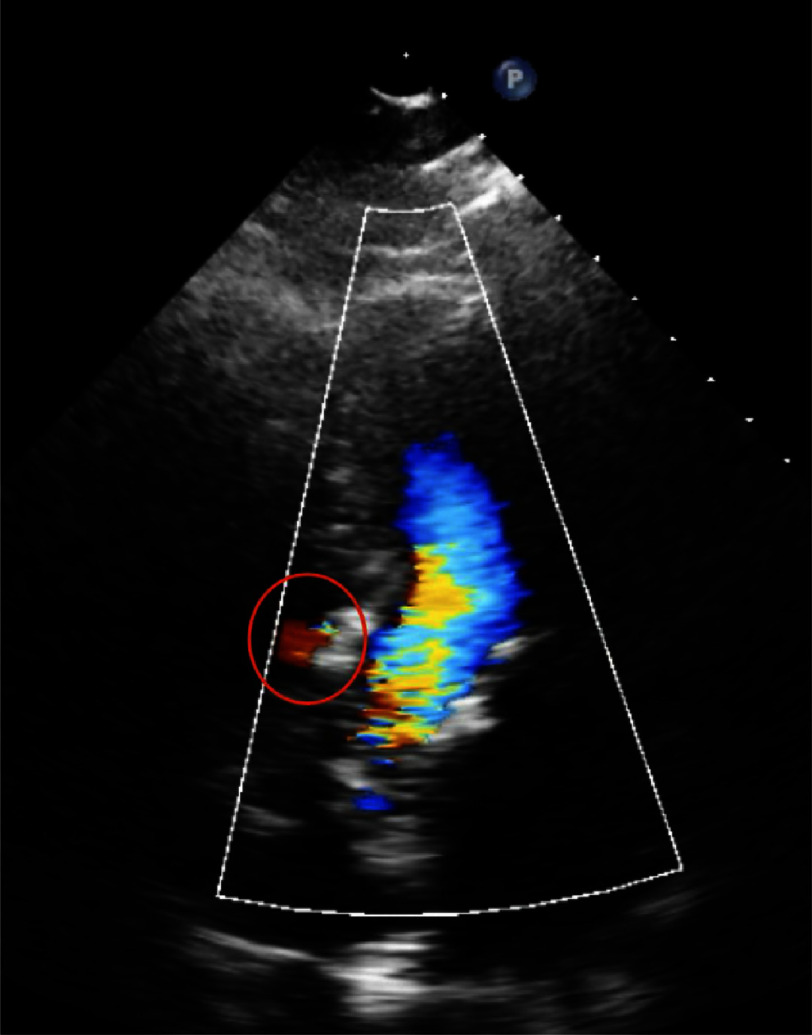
5-chamber view of transthoracic echocardiogram demonstrating membranous ventricular septal defect.
